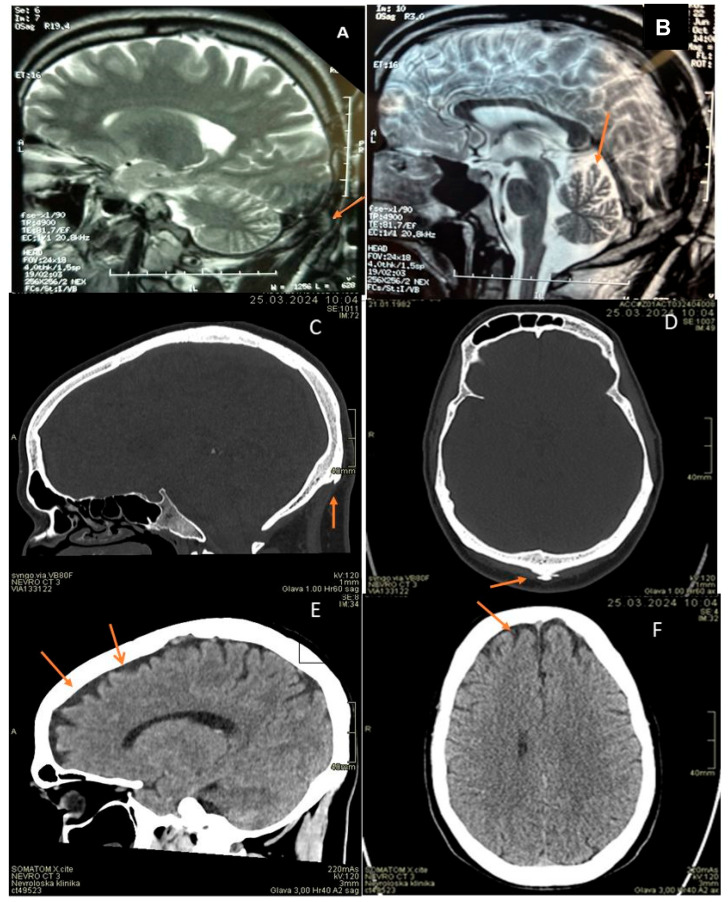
(A): patient 1, brain MRI, T2 sagital image: occipital horn (orange arrow). (B): patient 1, brain MRI, T2 sagittal image: dilated frontoparietal and cerebellar sulci (orange arrow). (C,D): patient 2, head CT, bone window, sagital and axial planes: occipital horn (orange arrows). (E,F): patient 2, head CT, soft tissue window, sagittal and axial planes. Mildly dilated frontal sulci (orange arrows).
